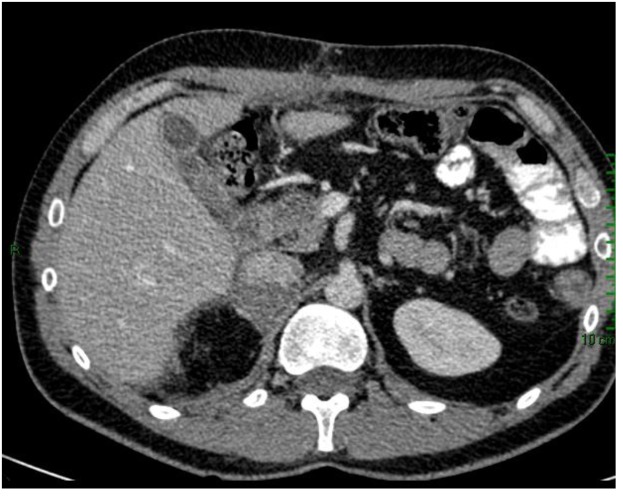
CT scan after surgical oncology showing right radical adrenalectomy and total omentectomy.
